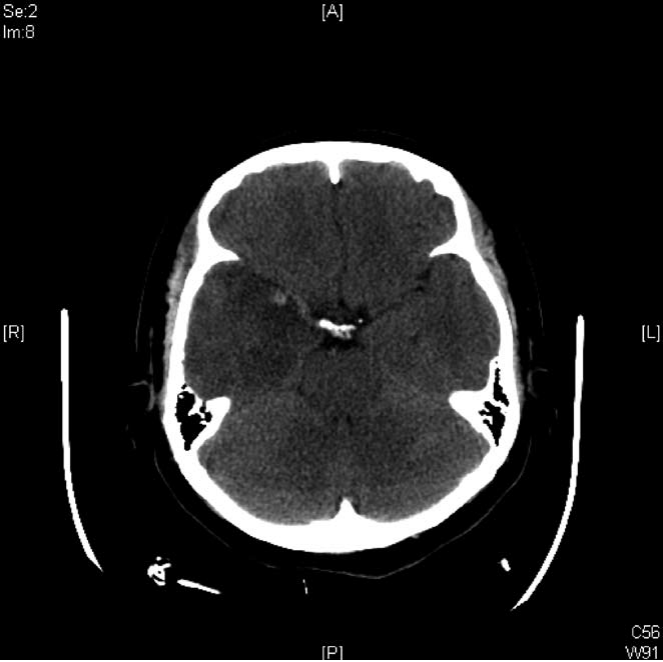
Computed tomography scan showing hemorrhage in edematous part of brain of patient with herpes simplex virus encephalitis, day 5 of hospitalization.
